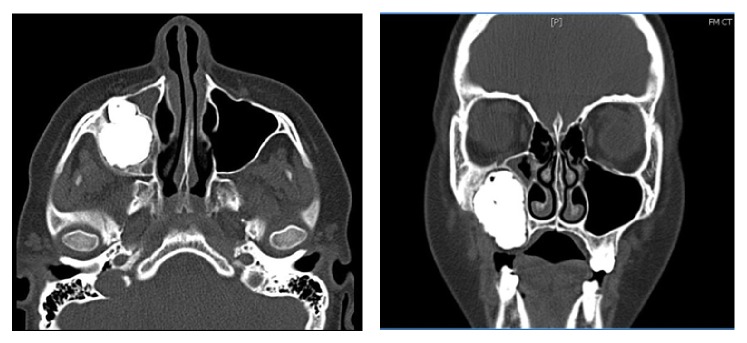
Axial and coronal CT images demonstrating the extent of the lesion in the right maxillary antrum.
